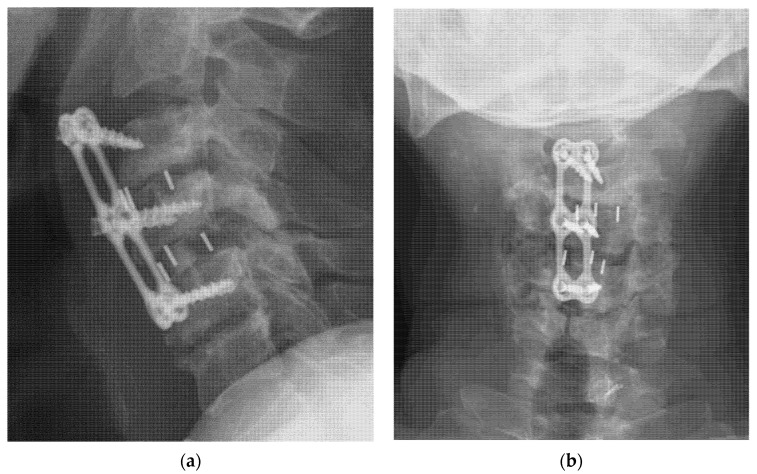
Radiograph after C3–4–5 ACDF surgery of a 50-year-old man with ESRD. (a) Lateral view and (b) anteroposterior view revealed implant dislocation with grade 4 fusion status at C3–4 and grade 3 at C4–5; grade 0 cage subsidence at both C3–4 and C4–5 levels without progression of neurological symptoms. ACDF, anterior cervical discectomy and fusion; ESRD, end-stage renal disease.
